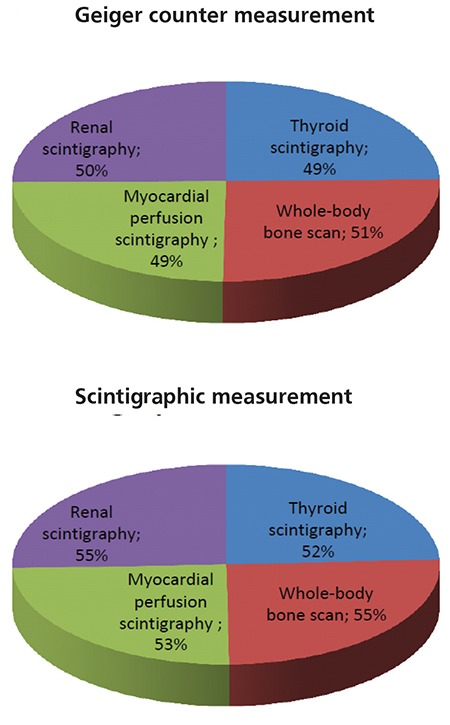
The percentage distribution of the total average decline ratiosin radiactivity according to the type of scintigraphic examinations for themeasurements obtained from Geiger counter and scintigraphic method
